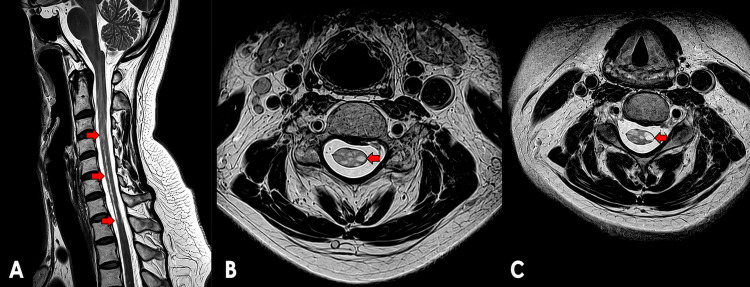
Follow-up cervical spine MRI obtained 3.5 months after symptom onset. (A) Sagittal T2-weighted image demonstrating persistent longitudinal non-enhancing intramedullary T2 hyperintensity extending from approximately C2 to T1 (red arrows) without significant interval change compared with the baseline MRI. (B-C) Representative axial T2-weighted images demonstrating persistent central and left-sided intramedullary T2 hyperintensity (red arrows). The lesions appear more well-defined on follow-up imaging, with radiologic features favoring chronic myelomalacic change.
